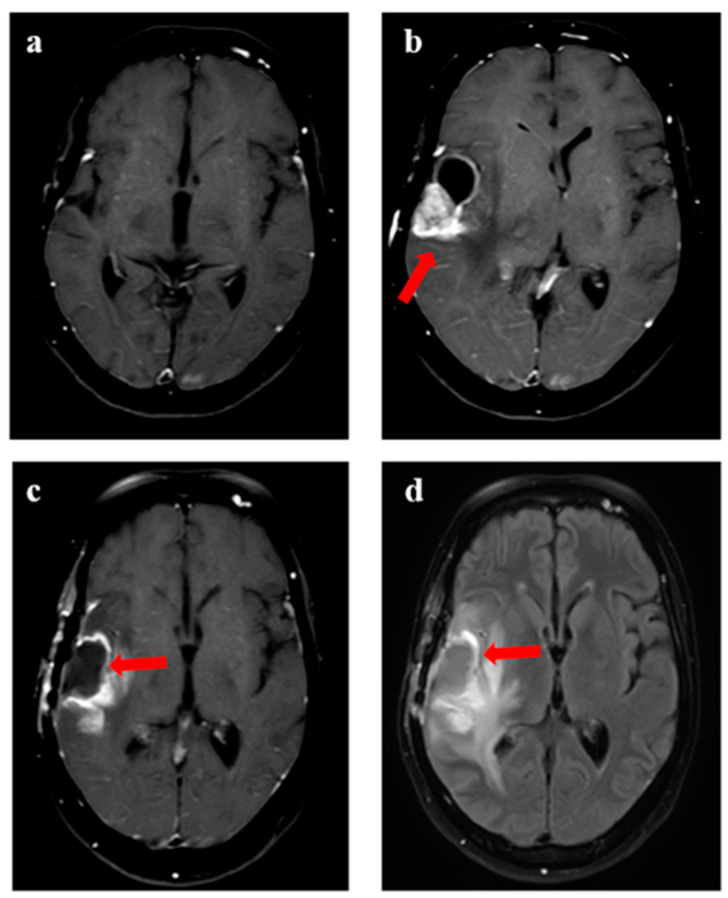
Progression in a 47-year female with glioblastoma with MGMT gene promoter methylation. (a) shows T1w axial view following completion of adjuvant chemotherapy (after surgery and radiation), with (b) showing local recurrence after 2.5 years with enhancing component and cystic component (arrow). (c,d) represented the surgical cavity on T1w contrast and T2w FLAIR axial view when the patient was considered for reirradiation.
